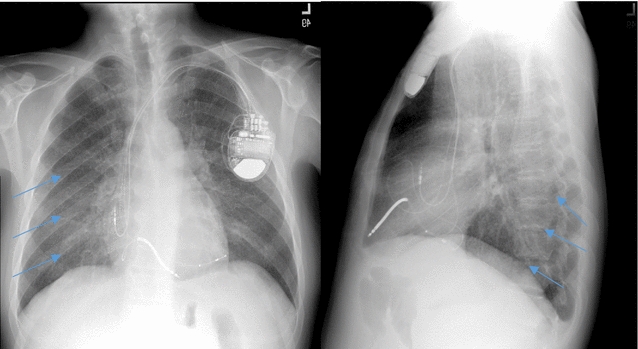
Posterior anterior and lateral chest X-ray showing diffuse miliary shadowing in both lungs (*Arrows*). The cardiac resynchronization therapy-defibrillator device and leads in place
